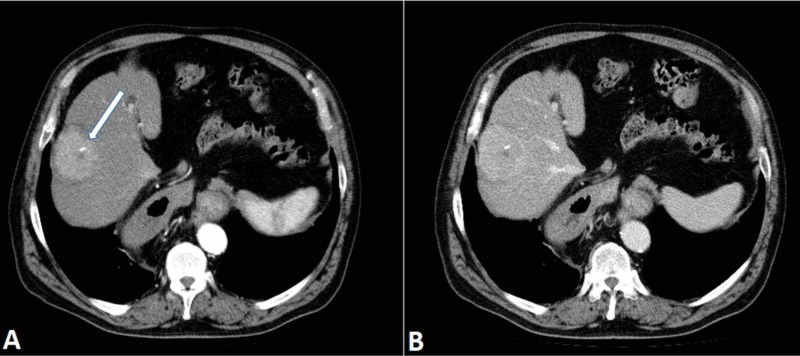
Liver mass. Axial CT scan in the arterial phase (A) demonstrates a mildly hyperdense mass in the sixth liver segment with a central calcification (arrow). The mass shows early arterial enhancement, but a typically fast wash-out characteristic for HCC is not seen (B). HCC, hepatocellular carcinoma
